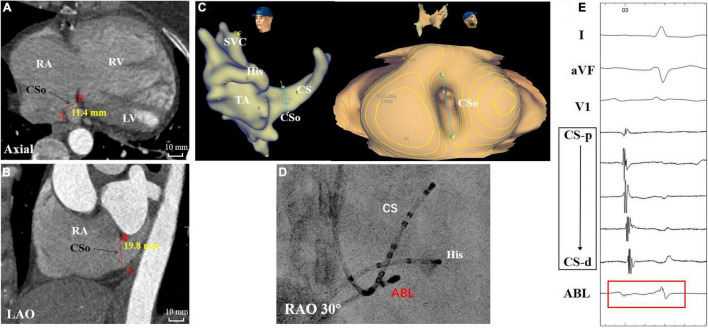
A patient with PAH and AVNRT showed marked vertical dilation of the CSo. (A,B) Measurements in the axial and LAO planes by CTA. (C) Three-dimensional image and panoramic view of the CSo. (D) Fluoroscopy of the ablation target. (E) Intracardiac electrogram of the ablation target (red box) during sinus rhythm. ABL, ablation catheter; AVNRT, atrioventricular nodal reentrant tachycardia; CTA, computed tomographic angiography; CS, coronary sinus; CS-d to CS-p: from distal-to-proximal pair of coronary sinus electrode; CSo, coronary sinus ostium; His, His bundle catheter; LAO, left anterior oblique; LV, left ventricle; PAH, pulmonary arterial hypertension; RA, right atrium; RAO, right anterior oblique; RV, right ventricle; SVC, superior vena cava; TA, tricuspid annulus.
